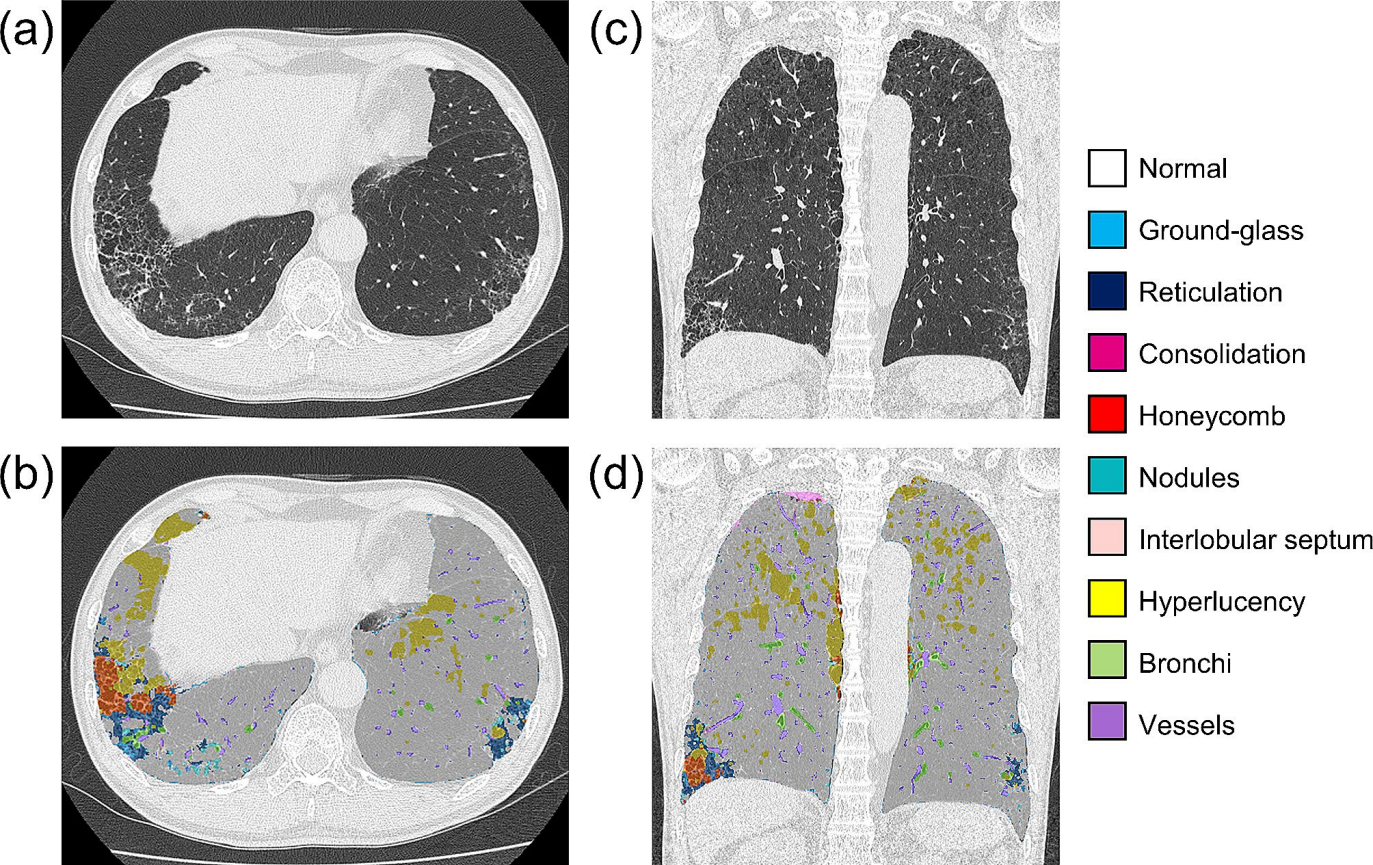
CT images of COPD with ILAs and corresponding AIQCT images Legend: The axial CT image of a 68-year-old male patient with COPD and ILAs in the discovery cohort (Fig. 2. a) and the corresponding AIQCT image overlaid on the original image (Fig. 2. b). Sagittal image of the same patient (Fig. 2. c) and the corresponding AIQCT image (Fig. 2. d). The ILD volume was 150 mL (2.90%)
